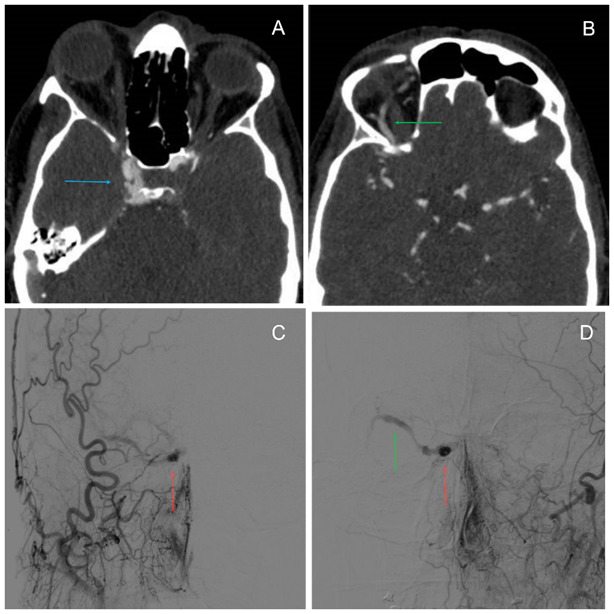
A. Angio-CT scan: in the artery phase, an image of early and abnormal filling of the right cavernous sinus (CS) was seen (blue arrow). B. Enlarged superior ophthalmic vein (SOV) characteristic of Carotid Cavernous Fistula (CCF) (green arrow). C and D. Brain arteriography revealed the abnormal filling of the right CS (red arrow) and enlarged SOV (green arrow) from right and left internal carotid artery respectively. Carotid Cavernous Fistula Type B Barrow Classification
